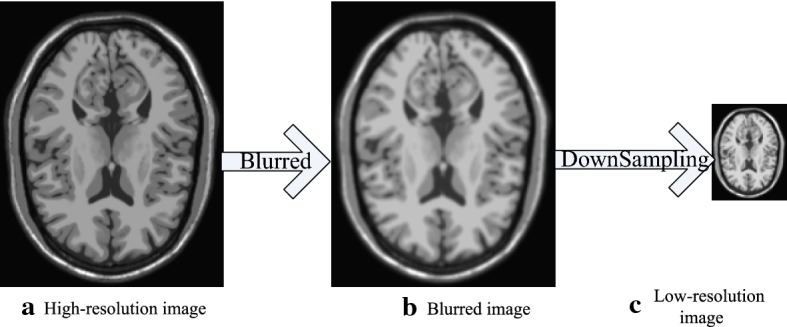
Degradation model for MRI
